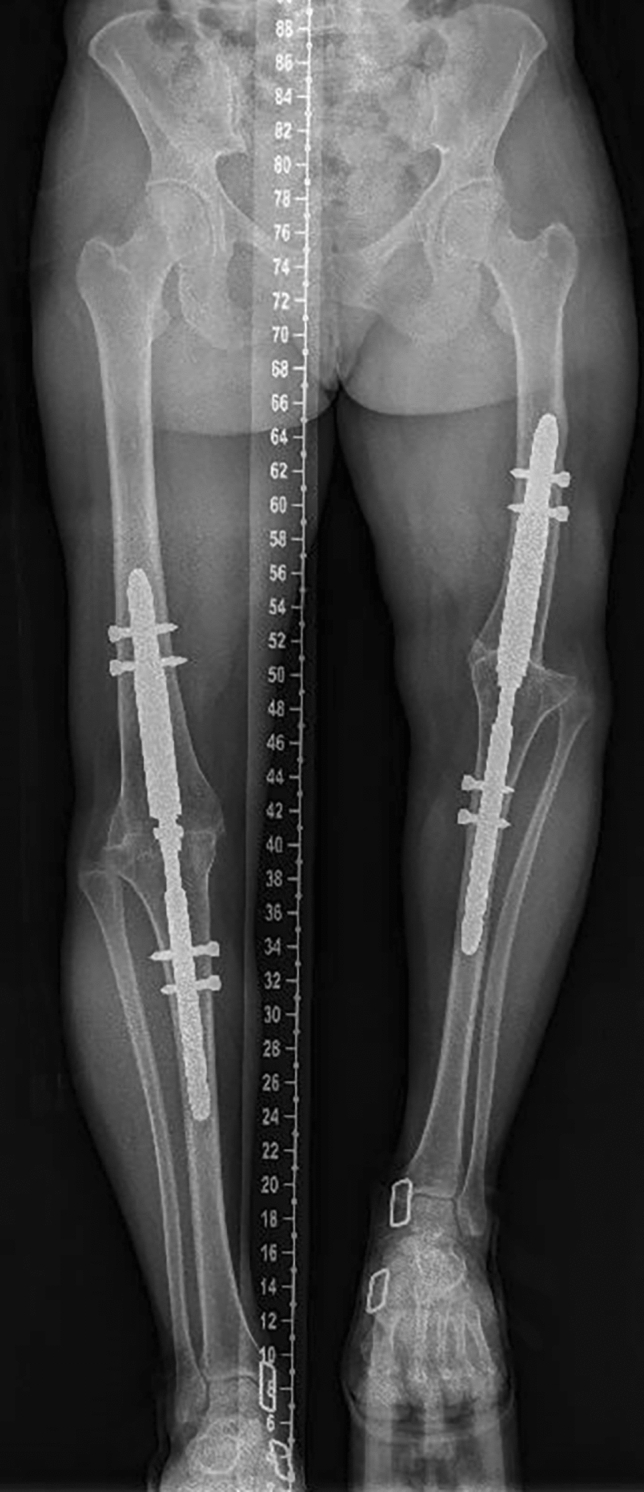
Standing whole leg radiograph of bilateral KA using WFN at 1 year
